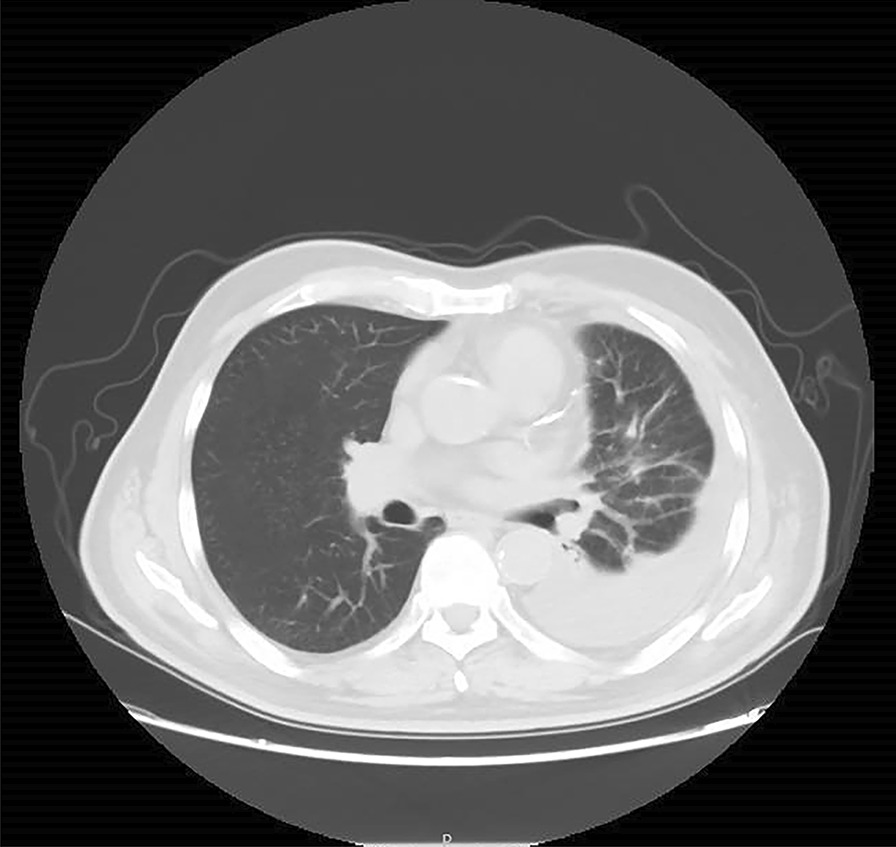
CT of the lung: obvious effusion signs were seen in the left thoracic cavity, patchy consolidation shadows were seen in the left lower lobe with incomplete swelling
